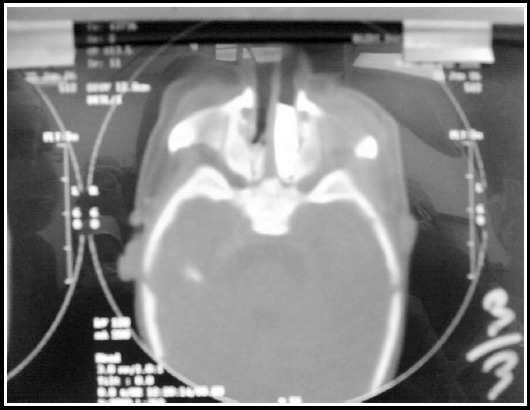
CT scan of the patient shown obstruction on both side of choanae.
